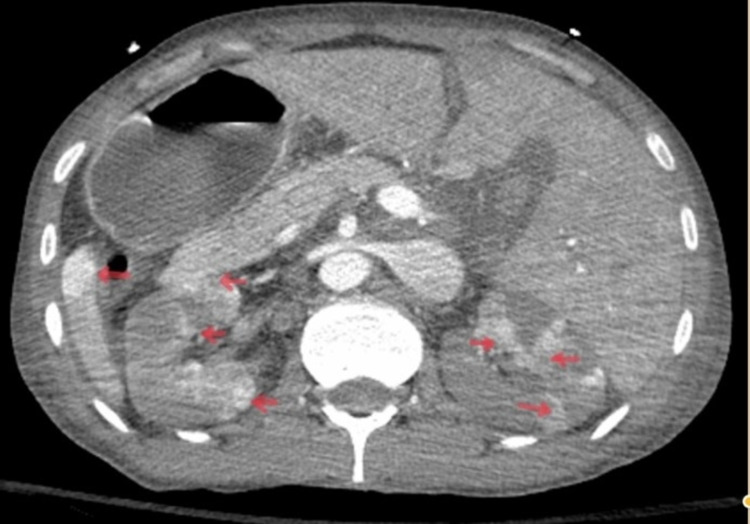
Computed tomography (CT) of abdomen/pelvis with contrast showing diffuse bilateral renal and splenic infarcts (arrows)
